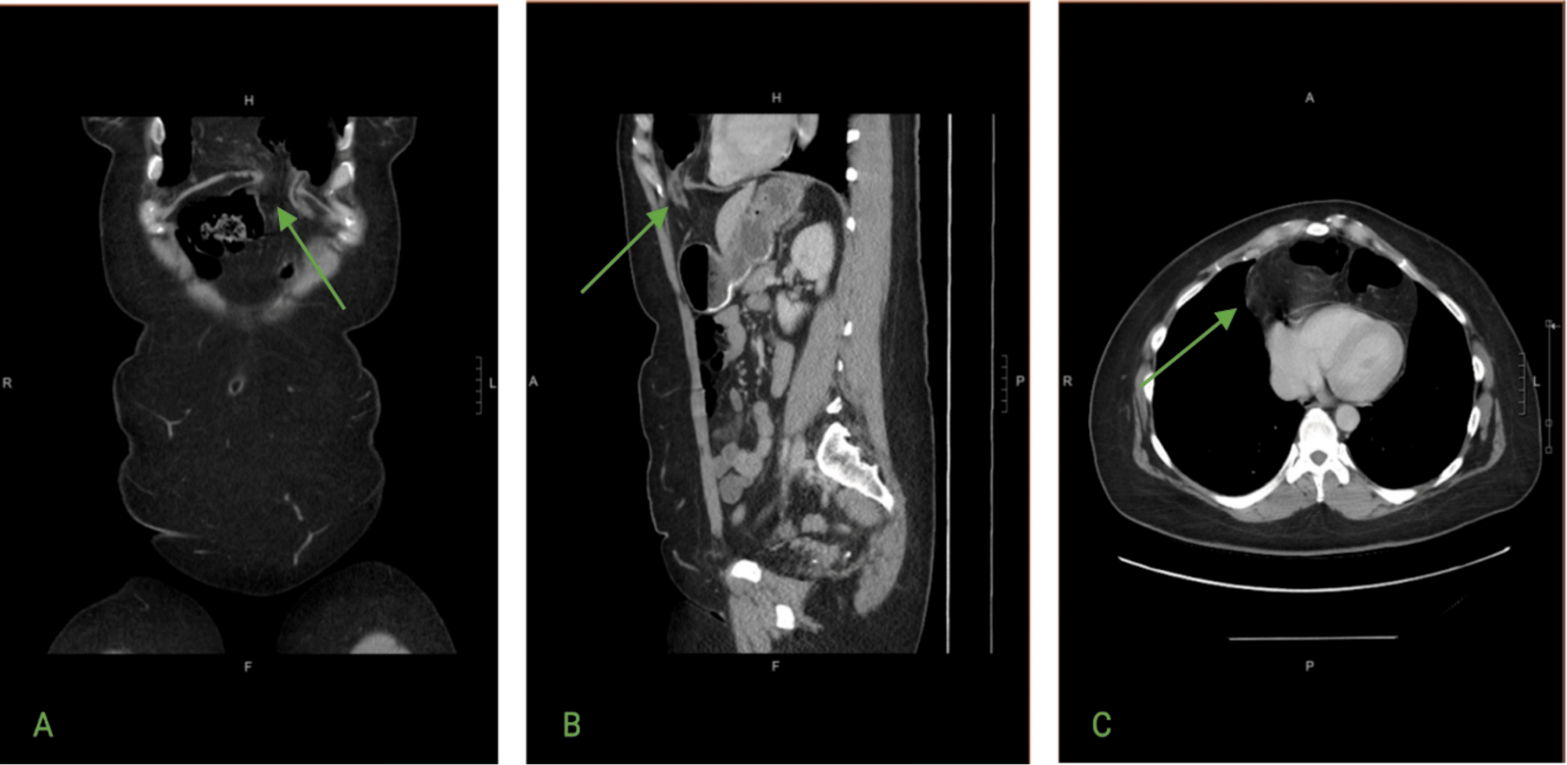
Computed tomography imaging of a Larrey hernia. (A) Coronal CT view showing a left-sided anterior diaphragmatic defect with herniation of abdominal contents into the thoracic cavity (green arrow). (B) Sagittal CT view demonstrating the herniated bowel loops passing through the diaphragmatic defect into the left thoracic cavity (green arrow). No evidence of significant mediastinal shift or compression of adjacent thoracic structures is observed. (C) Axial CT image showing a left-sided anterior diaphragmatic defect with herniation of abdominal contents (green arrow). The defect is consistent with a Larrey hernia.
